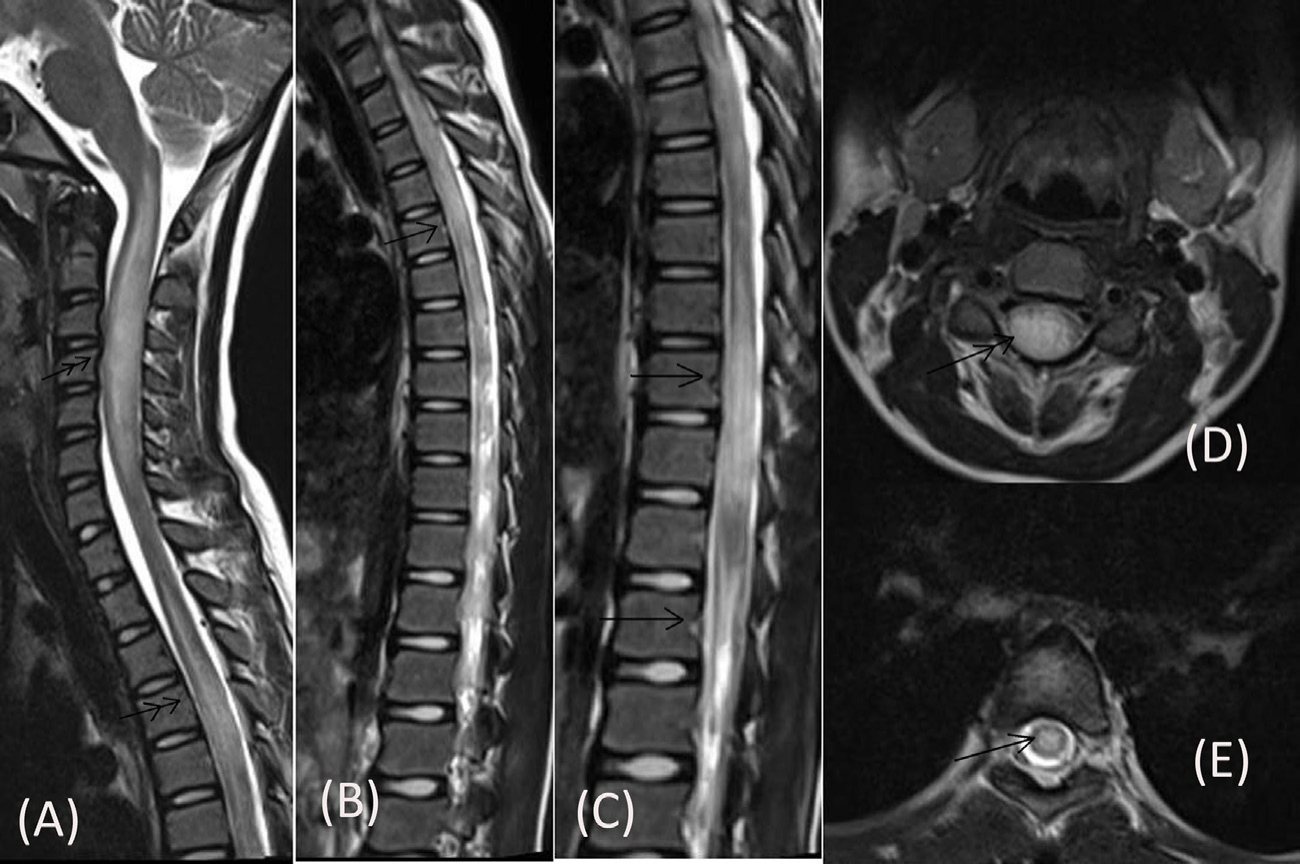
MRI of cervical spine done prior to admission showing long segment centrally located lesions in form of T2 hyperintensity in cervical cord (double arrow) [Saggital (A), Axial (D)] and dorsal cord (single arrow) [Saggital (B,C), Axial (E)].
